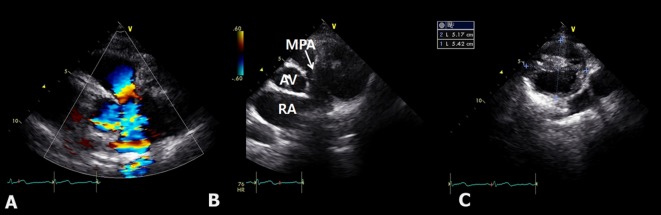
Transthoracic echocardiography demonstrating a turbulent color Doppler signal in the pulmonary trunk. (A) Compressing mass in the pulmonary trunk in the parasternal short axis view (B) multilocular cystic mass (C). RA: right atrium; MPA: main pulmonary artery; AV: aortic valve.
